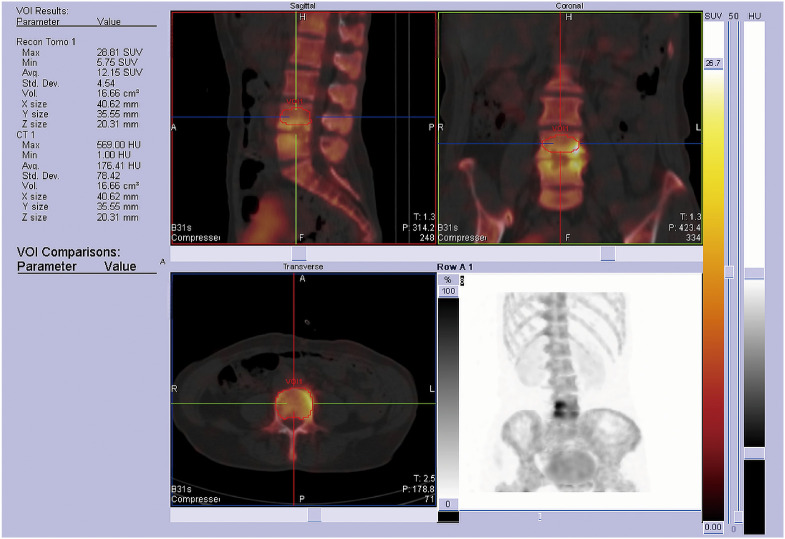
Quantitative single-photon emission computed tomography/computed tomography (SPECT/CT) examination obtained in a patient with spinal tuberculosis involving the L4 and L5 vertebra. Fused SPECT/CT images in the sagittal, coronal, and axial planes, together with the corresponding planar bone scintigraphy image, demonstrate increased radiotracer uptake in the involved L4 and L5 vertebral lesion.
